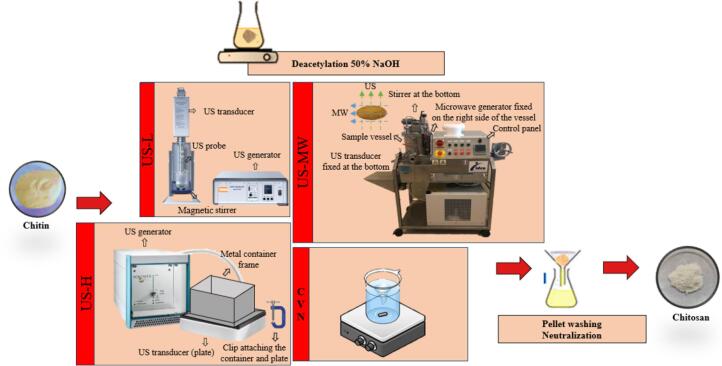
Chitin deacetylation to chitosan by conventional extraction (CVN), low-frequency ultrasound probe (US-L), high-frequency ultrasound plate (US-H), and ultrasound-microwave combination reactor (US-MW). US is ultrasound; MW is microwave.
